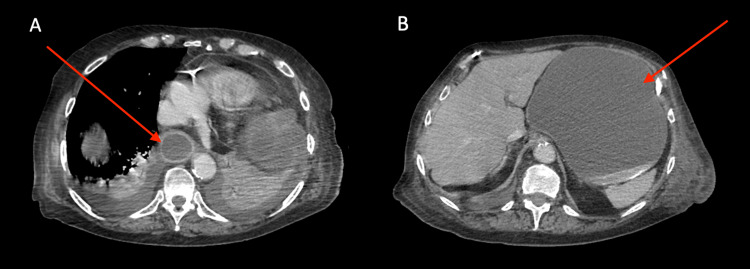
Computed Tomography of the Abdomen and Pelvis revealing distended viscera A - Dilated, fluid-filled distal oesophagus B - Dilated, fluid-filled stomach
